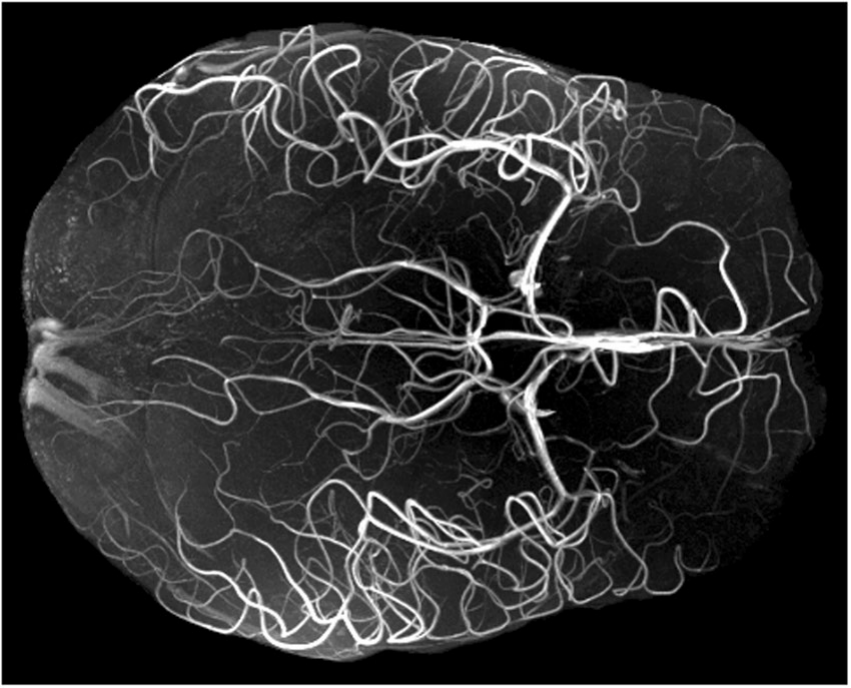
MRI data of the human arterial system. 3D model rotation can be controlled through the web interface and/or mobile device touchscreen.
